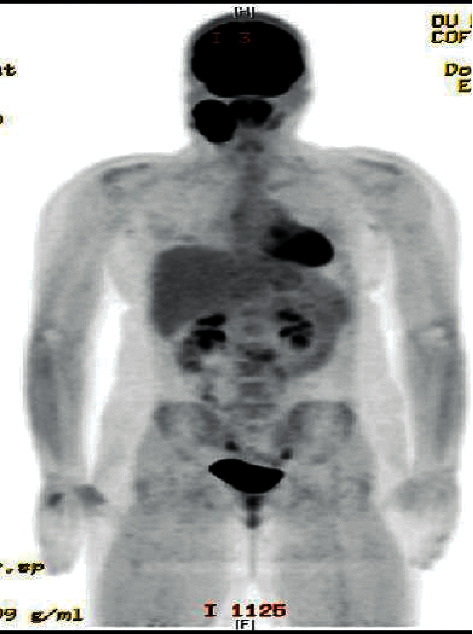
PET scan from a 17-year-old female showing a large FDG-avid right cervical mass, compatible with primary malignancy. No FDG-avid malignancy is identified elsewhere in the body.
